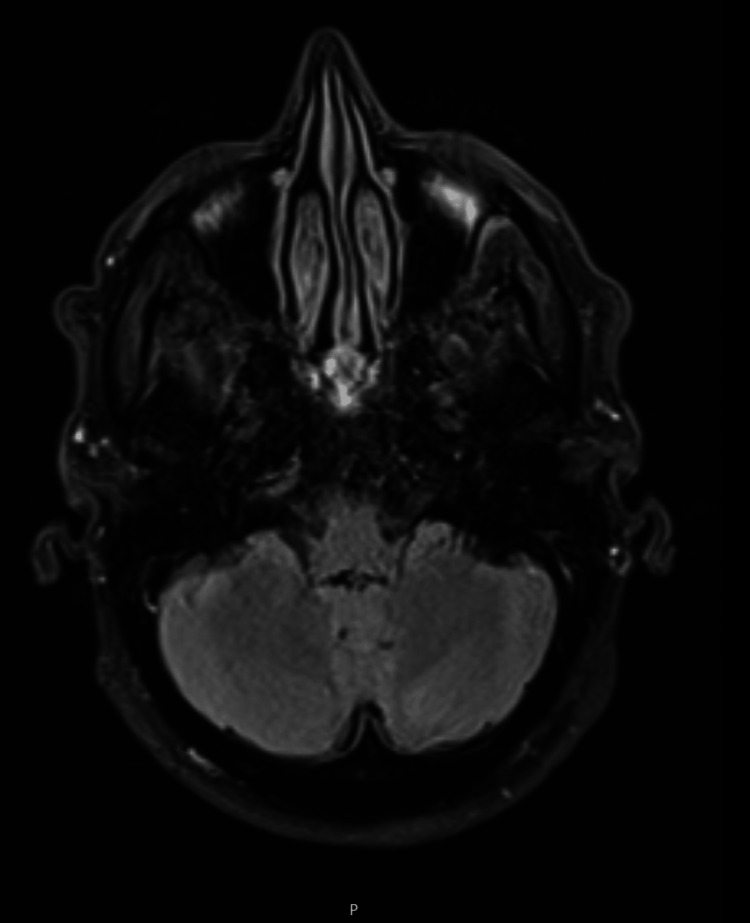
MRI brain: diffusion-weighted images with no evidence of diffusion restriction and no significant white matter abnormality.
